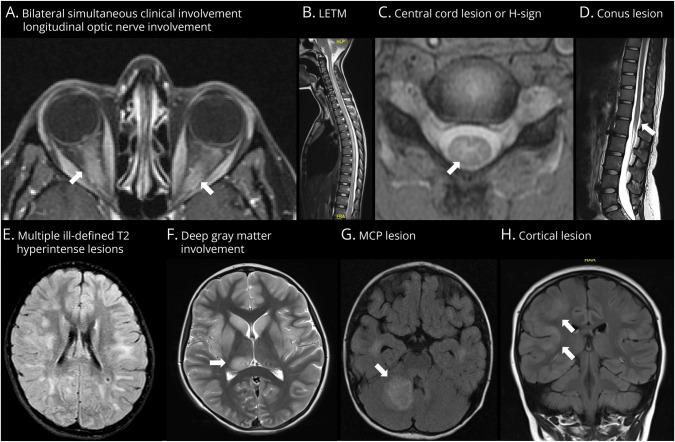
Radiologic Supporting Features (A) Axial gadolinium-enhanced fat sat T1-weighted scan of the brain showing longitudinal optic nerve involvement and bilateral simultaneous optic nerve involvement, which were the 2 most common supporting features in patients with predominant optic neuritis phenotypes. (B) Sagittal T2-weighted spinal cord scan showing a longitudinally extensive lesion in a patient with a predominant transverse myelitis phenotype. (C) Axial gradient echo T2-weighted scan of the cord showing the central lesion with a H-sign. (D) Sagittal T2-weighted spinal cord scan showing a conus lesion. (E) Axial FLAIR T2-weighted scan of the brain showing ill-defined lesions in a patient with brain, brainstem, or cerebral syndrome. (F) Axial T2-weighted scan of the brain showing a right thalamic lesion. (G) Axial FLAIR T2-weighted scan showing a lesion in the right middle cerebellar peduncle. (H) Coronal FLAIR T2-weighted scan demonstrating confluent hyperintensity of the right frontotemporal cortex. FLAIR = fluid-attenuated inversion recovery.
